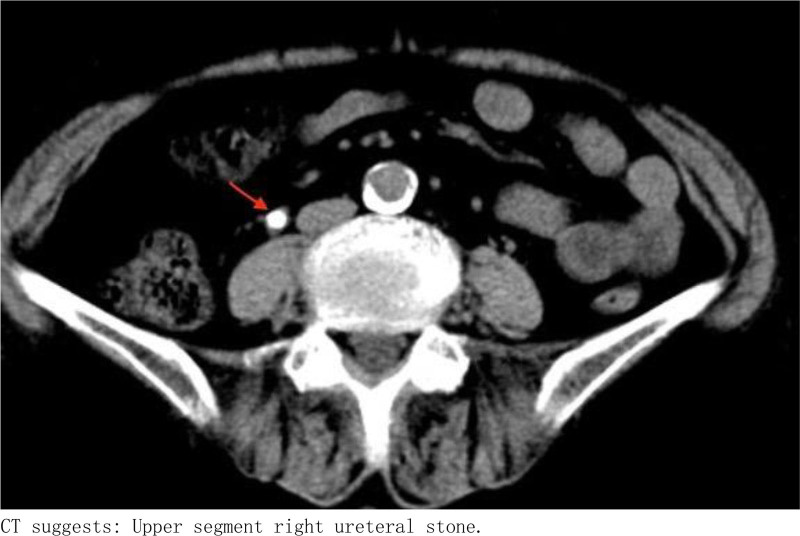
CT suggests: upper segment right ureteral stone. CT = computed tomography.
